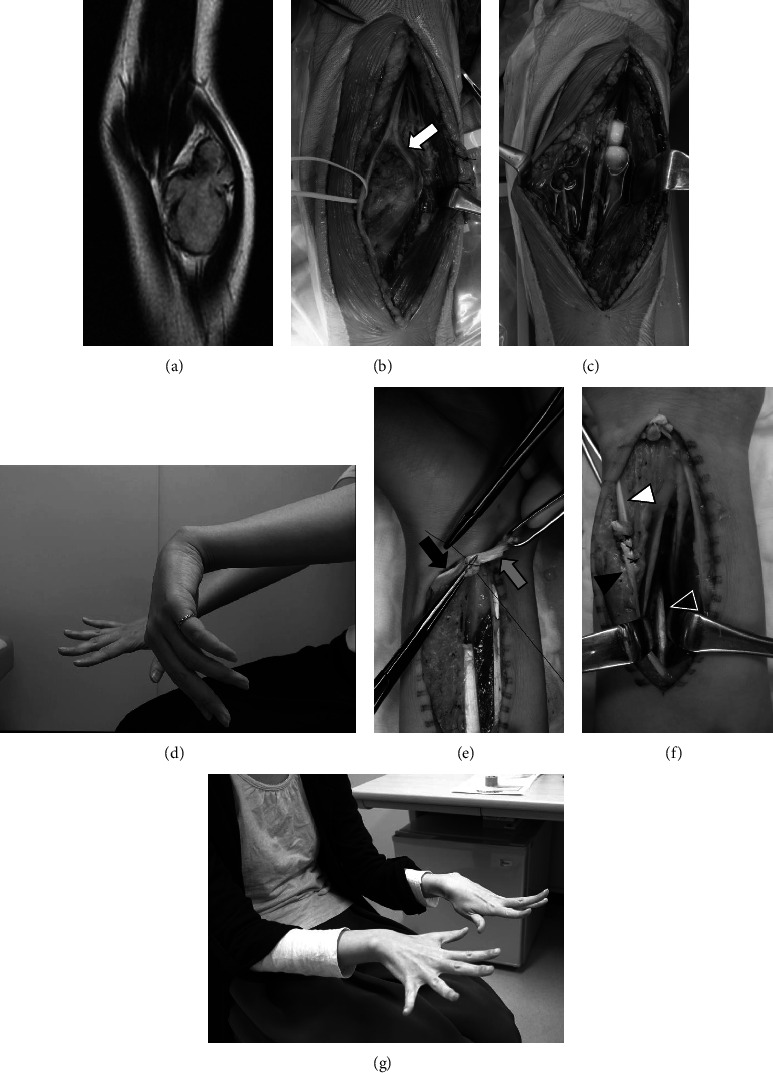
A 23-year-old female with a synovial sarcoma on the left elbow joint (case no. 8). MRI shows the iso-intensity tumor (53 × 32 mm) on T2WI (a). The posterior intraosseous nerve is involved in the tumor (white arrow) (b). The wide excision is performed and combined excision with joint capsule and posterior intraosseous nerve (PIN) (c). The patient shows the PIN palsy after 2 years (d). The tendon transfer (tsuge method) is performed 2 years after the initial surgery. The flexor carpi radialis (FCR) (grey arrow) is transferred to the abductor pollicis longus (black arrow) (e). The palmaris longus (black arrowhead) is transferred to the extensor pollicis longus (white arrowhead) and the FCR (white line arrowhead) is transferred to the extensor digitorum communis (f). The patient demonstrates full extension of fingers 2 years after the second surgery (g).
